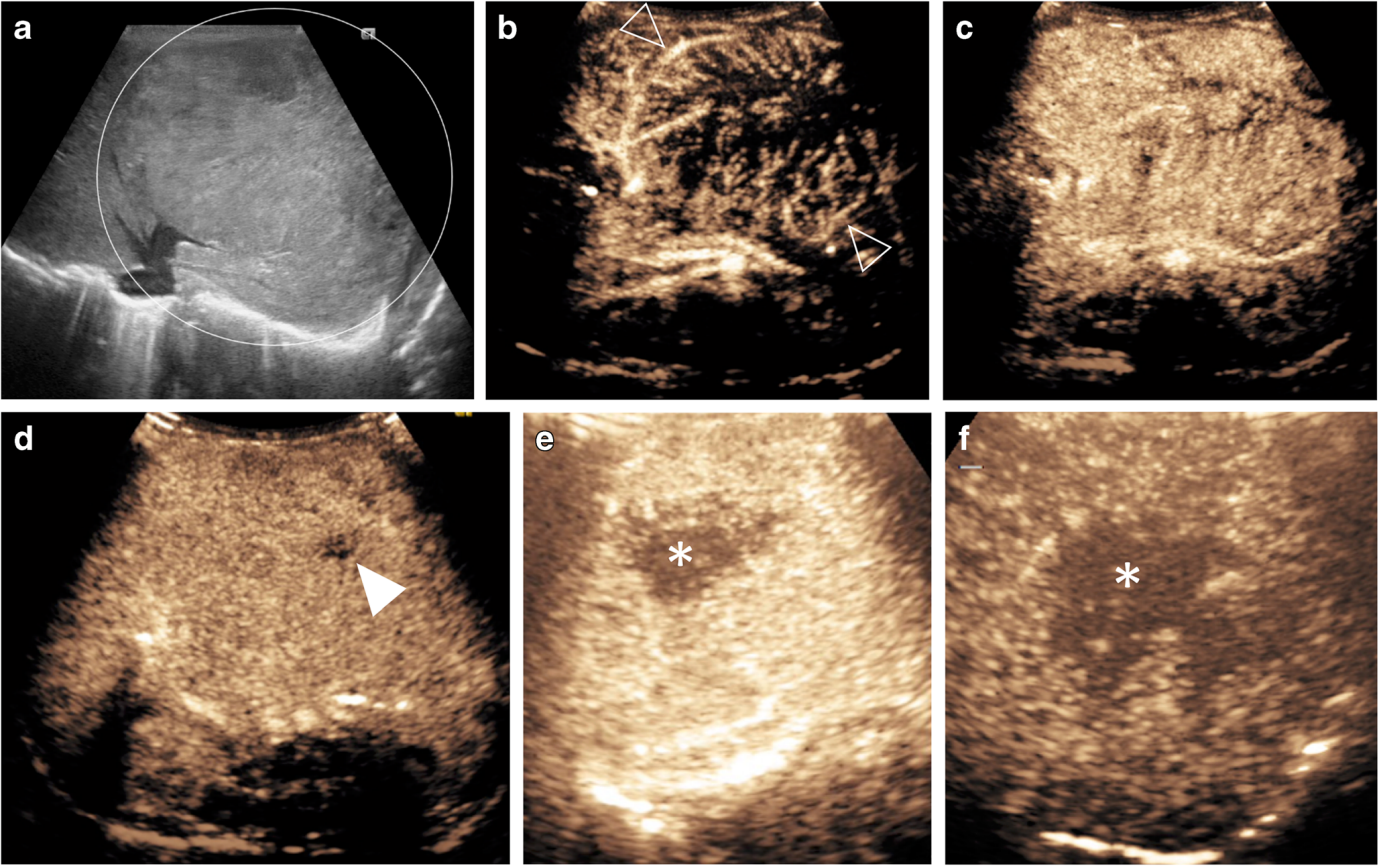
Hepatoblastoma in an 11-day-old boy with a large congenital mass of the left liver lobe. a Gray-scale US shows a large mass with heterogeneous echotexture (*circle*) within the left liver lobe. b–f Contrast-enhanced ultrasound (CEUS) of the liver. Transverse CEUS in contrast-only mode in arterial phase at 10 s (b) and 14 s (c), in portal venous phase at 53 s (d) and in late-phase of enhancement >120 s after contrast injection (e, f). Rapid heterogeneous enhancement shows multiple irregular vessels (*open arrowheads*) centrally within the lesion in a disorganized pattern. One small region in the periphery of the lesion (*solid arrowhead*) shows persistent reduced enhancement, possibly corresponding to necrosis. Transverse CEUS during the late phase (e and later f) shows progressive washout (*asterisks*) centrally
